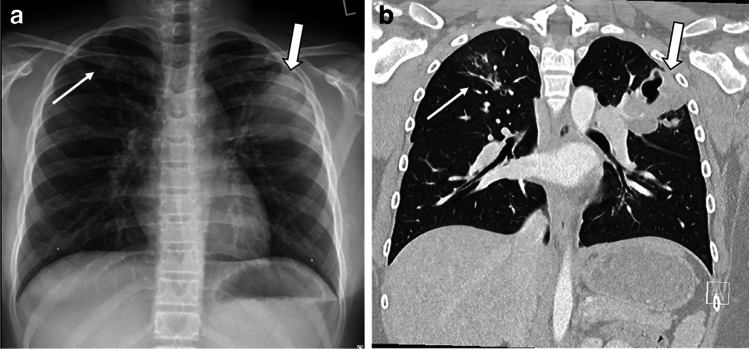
Acute pulmonary exacerbation in a 10-year-old boy. a, b Posteroanterior (PA) chest radiograph (a) and coronal end-inspiratory CT (b) show large cavitating consolidation in the left upper lobe (*thick arrow* in both). More subtle changes such as peribronchial opacities and mucus plugs in the right upper lobe are less visible on chest radiograph (*thin arrow* in a) compared to CT (*thin arrow* in b)
